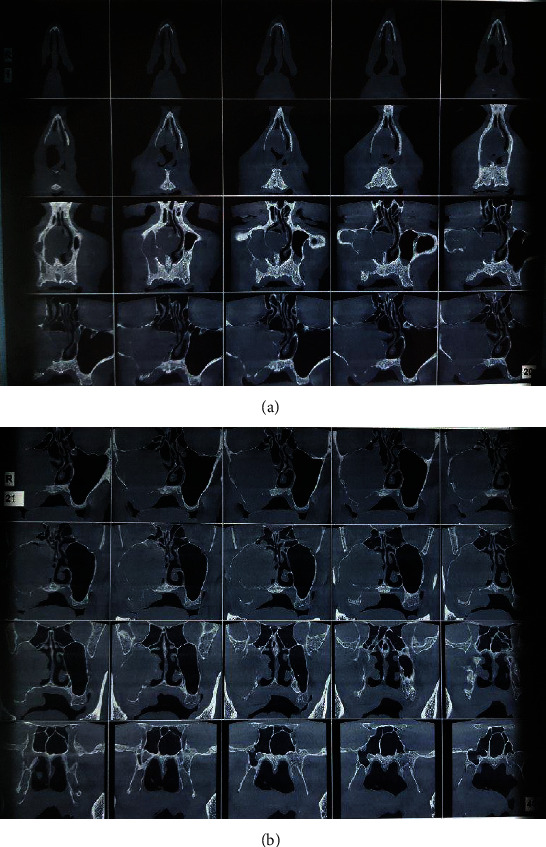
(a, b) Axial sections of cone-beam computed tomography (CBCT) presented a homogeneous hypodense lesion in the right maxillary sinus. The lesion has a destructive nature, destroying the buccal and lingual wall of the right alveolar process. In higher cuts, the lesion has destroyed the sinus's anterior, middle, and posterior lateral walls. The lesion has extended to the nasal cavity by destroying the medial and anterior walls of the sinus and presents as a mass of soft tissue in the nasal fossa. The periosteal reaction is not seen, and the pterygoid processes are intact. The sphenoid sinus is normal without any secretions, but the lateral wall of the right ethmoid sinus has been destroyed by the lesion. The lesion has caused nasal septum deviation.
